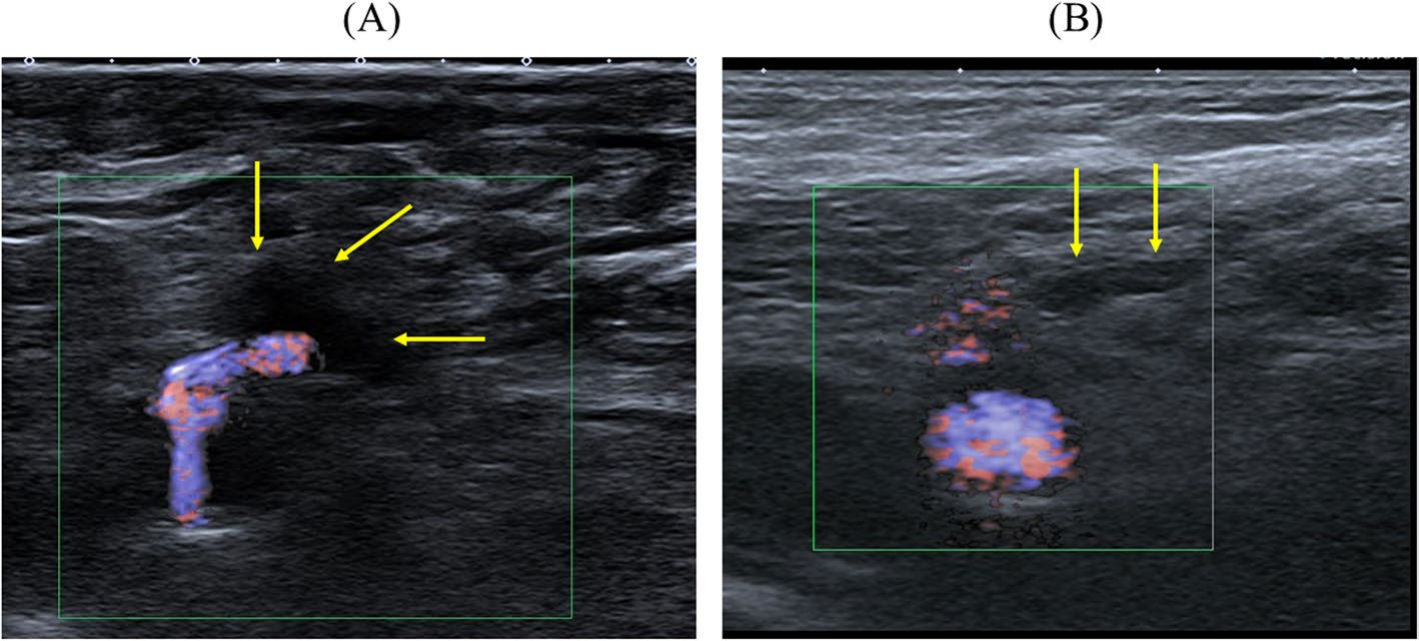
The ultrasonography images of the access site (A) inflow of blood from the puncture site into the hematoma (B) no blood flow into the hematoma was observed
